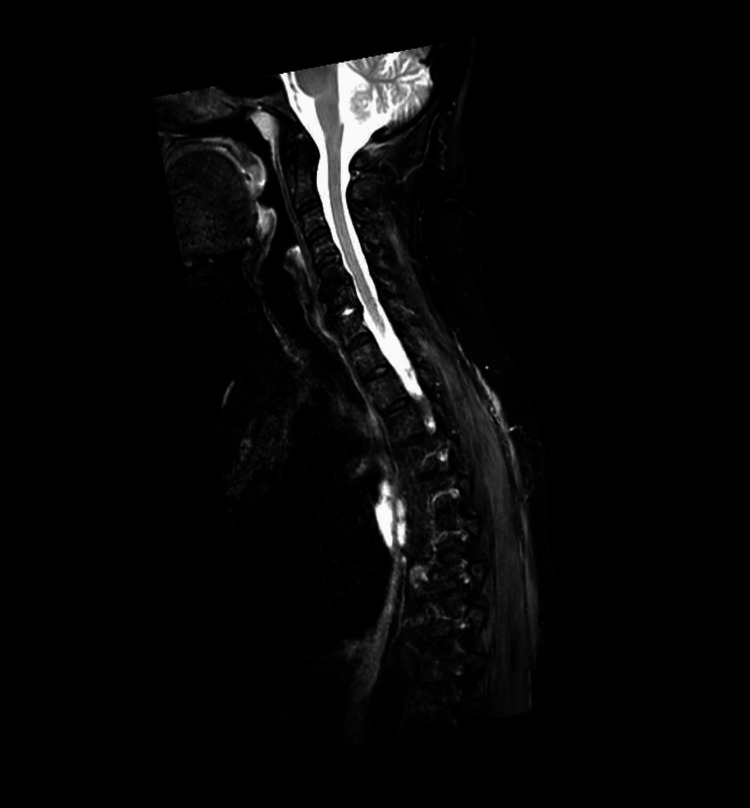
MRI of the cervico-thoracic spine (T2 sequence, sagittal view) showing para-spinal muscle oedema
